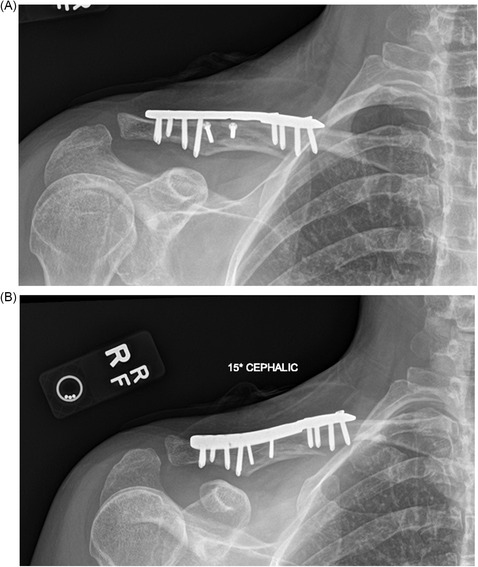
Right postoperative anteroposterior (AP) radiographs with no tilt (A) and with 15° cephalad tilt (B) that were taken 2 weeks status post clavicle ORIF with soft cerclage.
